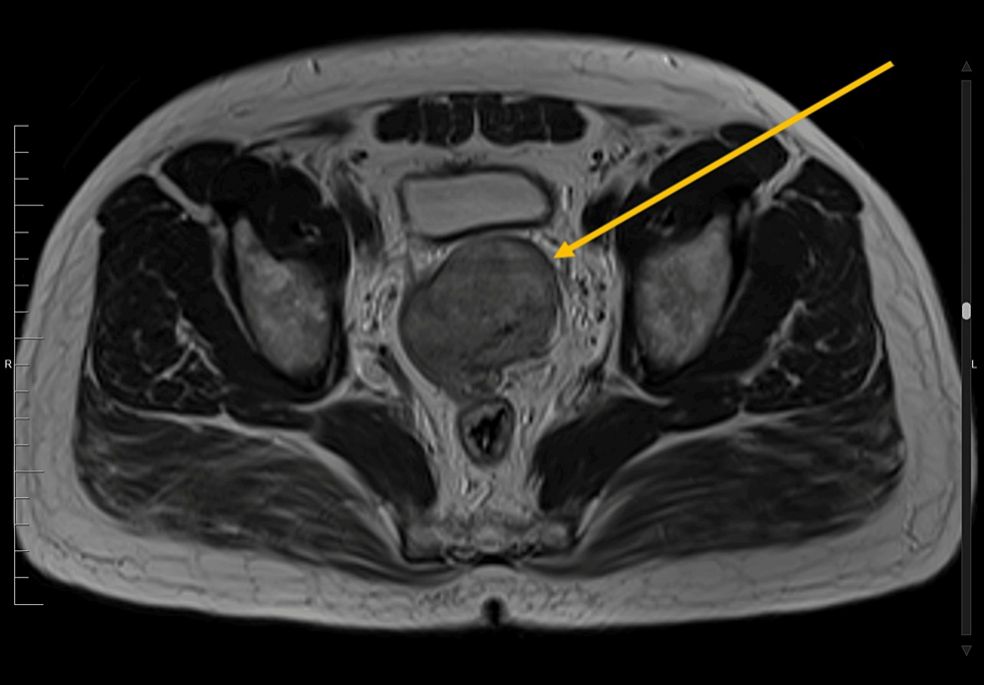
Abdominal MRI: T2-weighted image shows a T2 hyperintense mural rectosigmoid mass.
